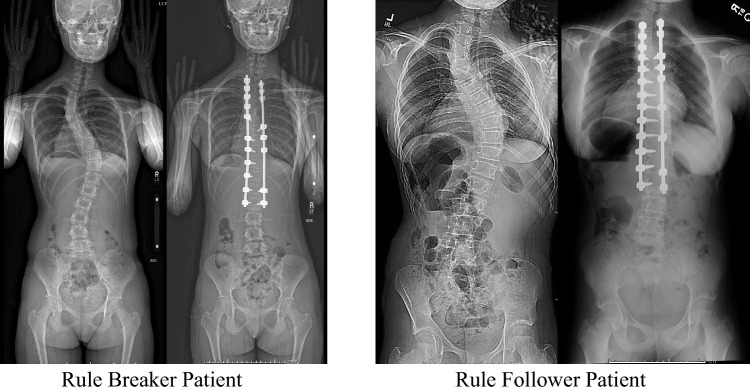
Preoperative and postoperative PA radiographs of Rule Breaker and Rule Follower showing that following current guidelines does not ensure improved shoulder balance
